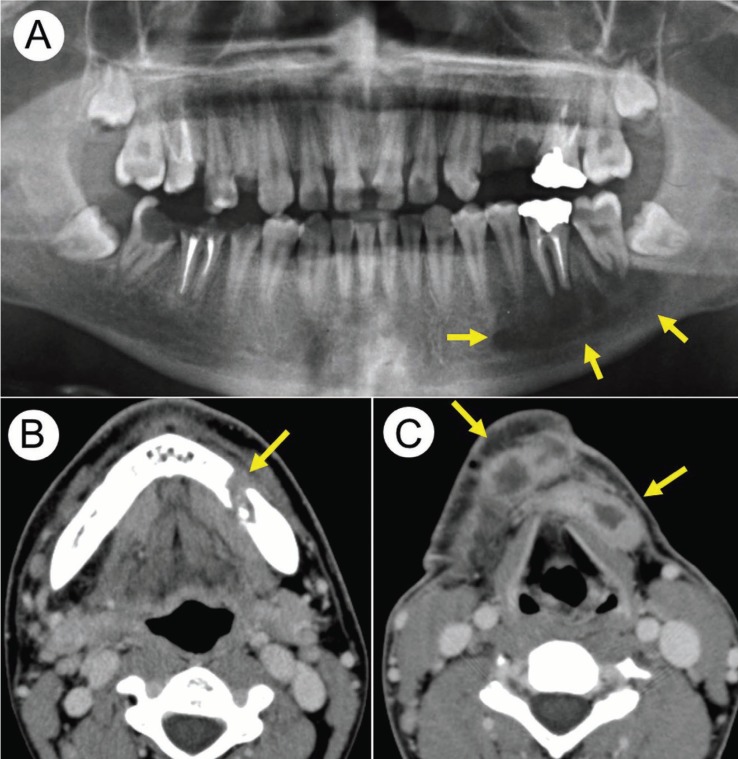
(A) Panoramic radiograph at the first visit (6 months preoperatively). (B) and (C) CT scan 1 week before cervical drainage and necrotomy.
